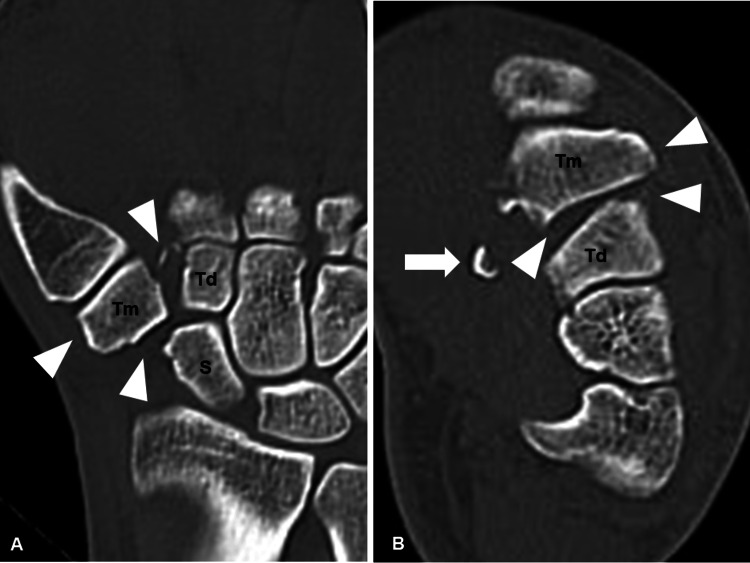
Preoperative plain computed tomography images. (A) Coronal and (B) axial reconstructed computed tomography images (arrowheads show dislocation of the trapezium, and. arrow show volar fragment of the trapezium). S, scaphoid; Td, trapezoid; Tm, trapezium
